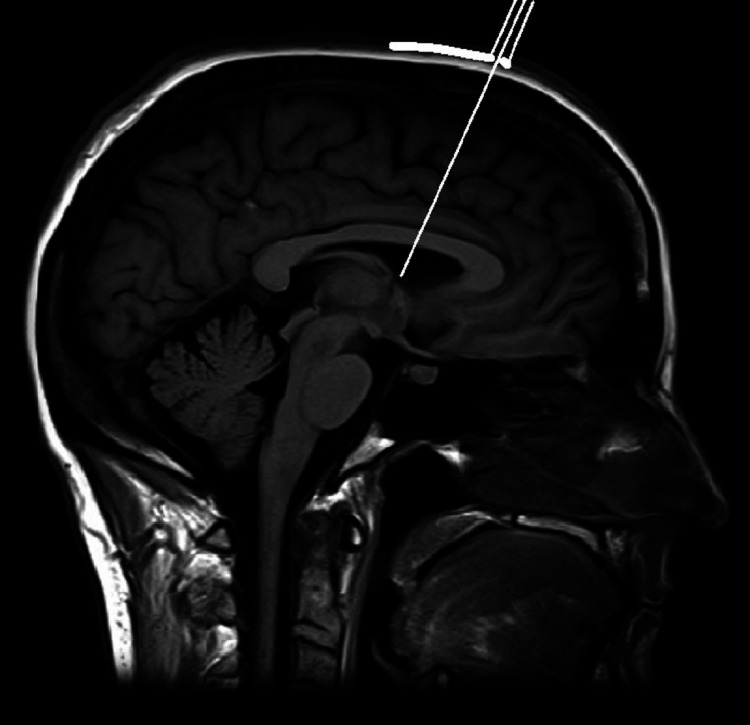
Shows the template of the scope on the top of the head on sagittal MR image with the probe holder aimed at the foramen of Monro
